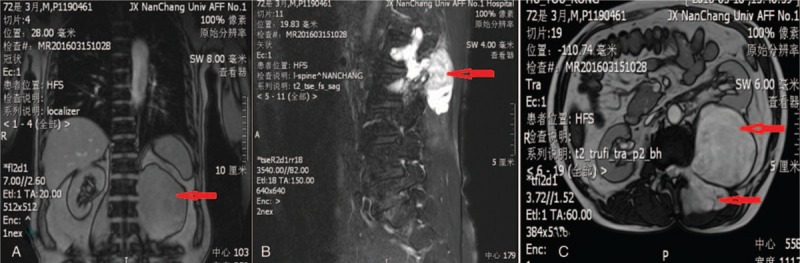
(A, B) Coronal and axial MRI revealed a large inhomogeneous mass causing the compression of the left kidney. (C) Sagittal MRI showed that the vertebral laminae of L2 was eroded and the left L2 intervertebral foramen was enlarged.
